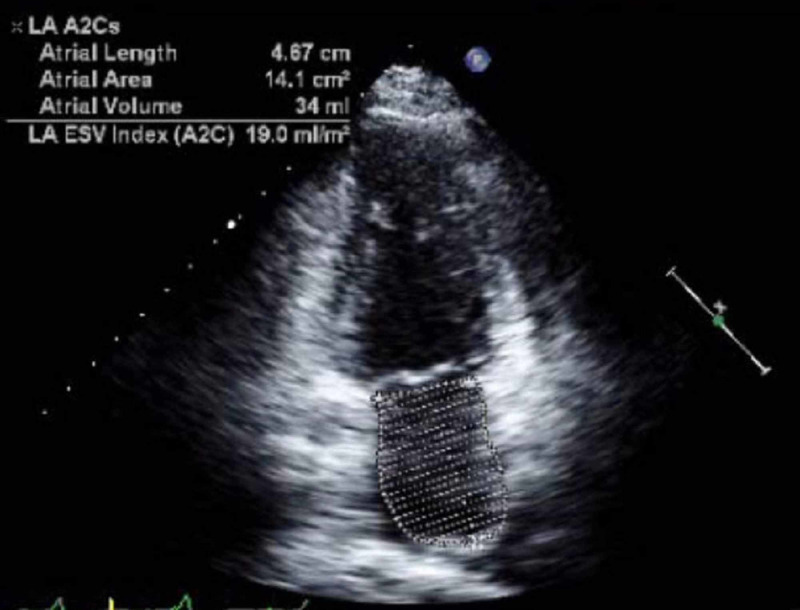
Echocardiogram. Dedicated apical two chamber view of left atrium and left ventricle shows moderate left atrial enlargement as evidenced on modified biplane method with calculated volume of 34 mL/m^2^
